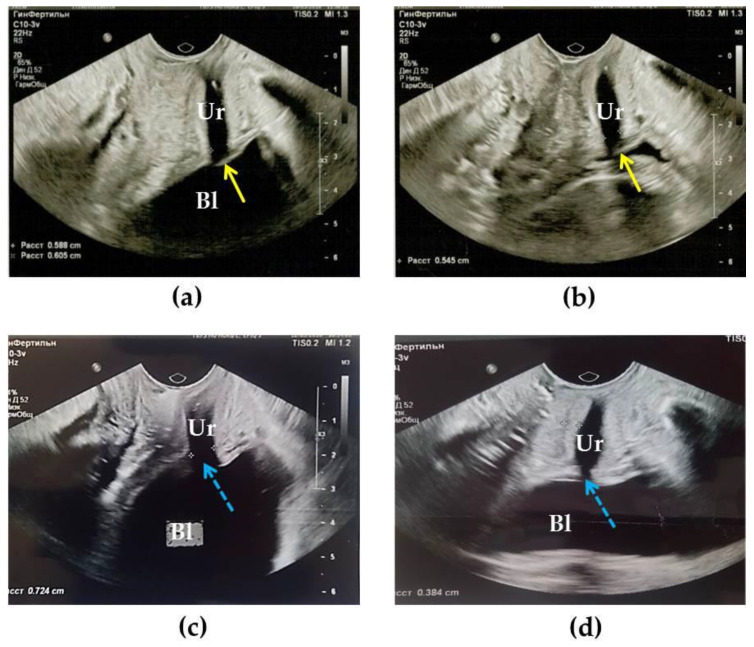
Transvaginal ultrasound (TVUS) of the urethra and adjacent tissues in normal conditions and with UPS. (a,b) A healthy woman 30 years of age before (a) and after (b) urination. The urethral tongue closes the opening to the urethra, as indicated by the yellow arrow; (c) Patient K., 30 years old, with a UPS disease duration of more than 10 years; (d) Patient Z., 38 years old, over 13 years of illness. In both cases, with UPS, the urethral tongue is indistinguishable and the gaping opening at the transition of the bladder into the urethra is indicated by the blue dashed arrows. Bl—bladder, Ur—urethra.
